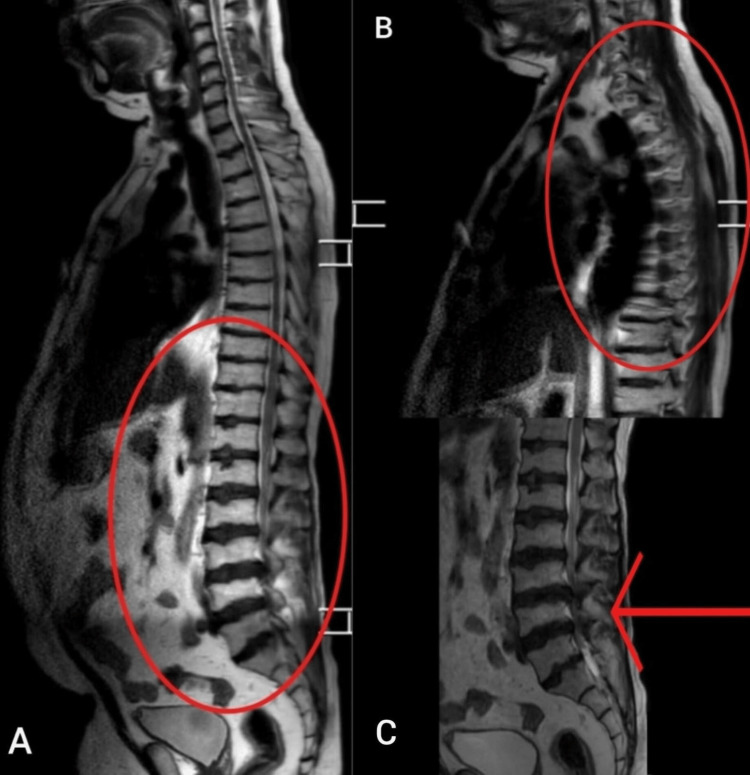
MRI of the lumbosacral spine (lateral view) MRI: magnetic resonance imaging
